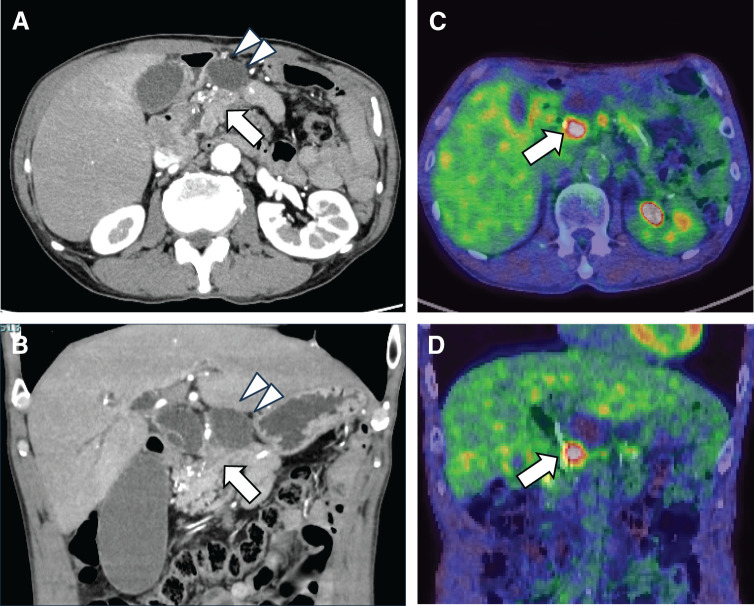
Preoperative imaging findings. Contrast-enhanced CT in the sagittal and coronal views (A, B) and PET-CT in the sagittal and coronal views (C, D) demonstrate a pancreatic head tumor (arrows) located adjacent to a pancreatic pseudocyst (arrowheads), accompanied by dilatation of the intrahepatic bile ducts. Increased fluorodeoxyglucose uptake is observed in the pancreatic head tumor.
